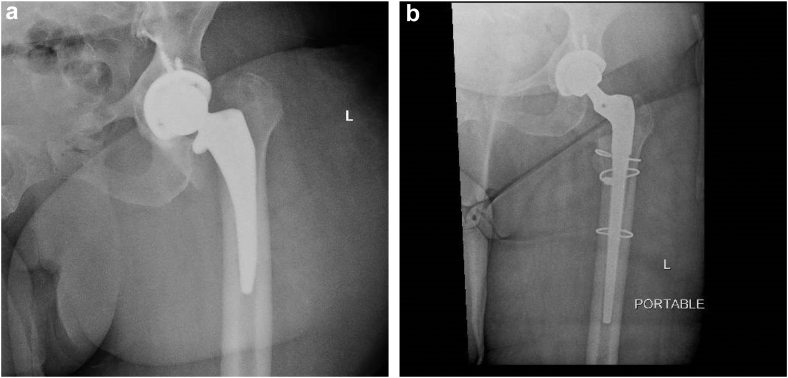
Radiographs of periprosthetic femur fracture and revision. Representative radiographs of a patient with periprosthetic femur fracture 1 month postoperation requiring revision. (a) An intertrochanteric periprosthetic fracture at the level of the implant with moderate displacement and comminution. (b) Postrevision radiographs with a new, long-stem revision prosthesis and 3 cerclage wires.
